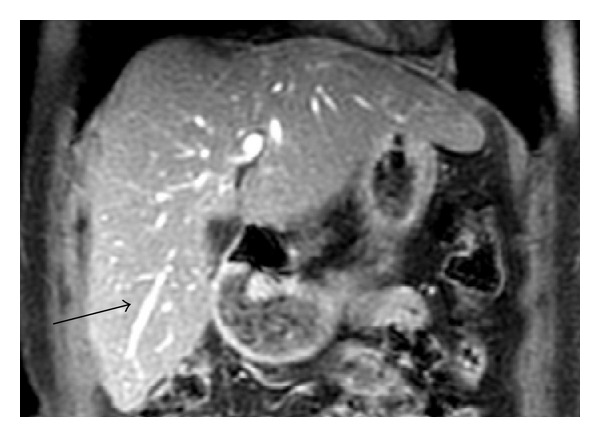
T1 weighted Magnetic Resonance image showing inferior right hepatic vein (arrow).
